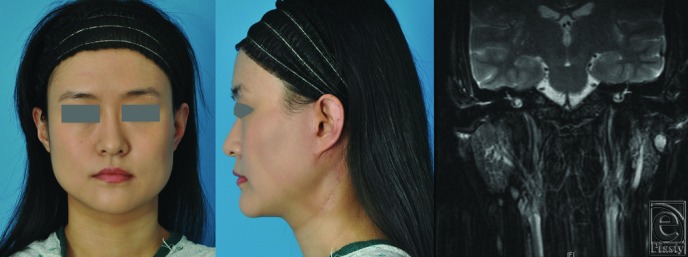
Preoperative presentation: (a) frontal view and (b) lateral view. (c) Magnetic resonance image demonstrating tumor within the left parotid gland.
